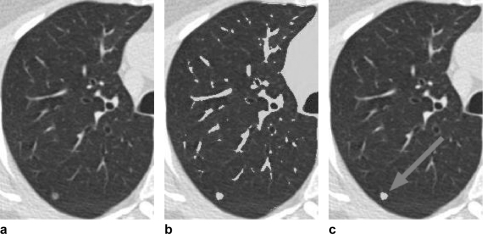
(a) Original CT image of the right lung. (b) Result of attenuation thresholding in the lung field, with ROIs corresponding to blood vessels and pulmonary nodule in gray. (c) Automatically detected nodule (gray with arrow) following classification step.
